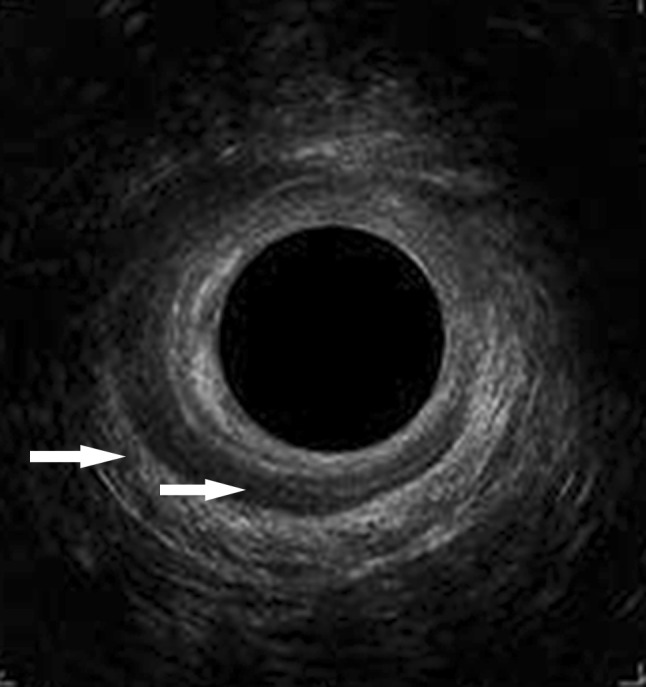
Endoanal ultrasound image. The hyperechoic outer circle is the external anal sphincter, and a defect is visible from the 9 o’clock position to the 3 o’clock position where the circle is discontinued and interrupted by a more hypoechoic area. The hypoechoic inner circle is the internal anal sphincter, and a defect is visible from the 11 o’clock position to the 4 o’clock position. Arrows point to each of the sphincters
